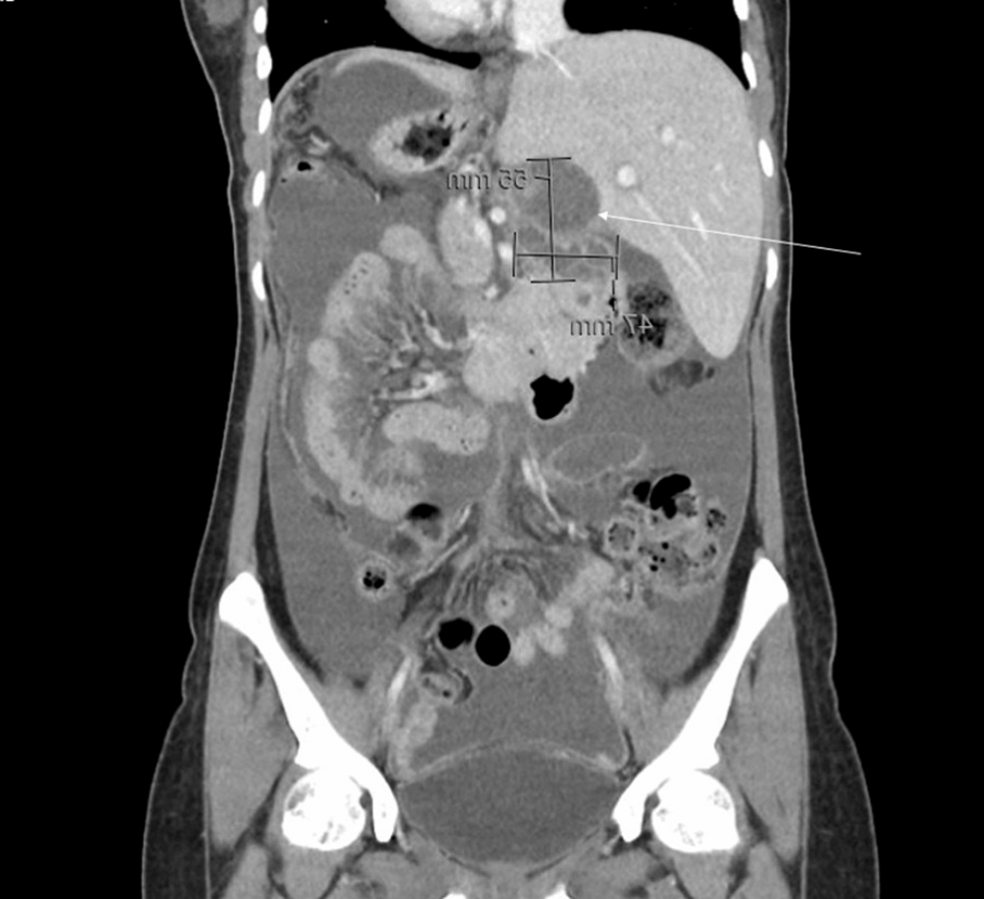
Coronal computed tomography image of the multiloculated cystic lesion (white arrow) as described in Figure 2
